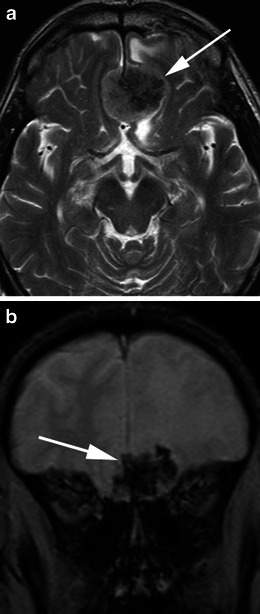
a Axial T2-weighted sequence in a 75-year-old man being investigated for Bell palsy demonstrates a heterogeneous, extra axial mass in the midline of the olfactory groove, left more than right, in keeping with a meningioma (*arrow*) associated with mild oedema within the left frontal lobe. There are intrinsic areas of low signal intensity in keeping with calcification that do not enhance on post contrast sequences. b Coronal T2* sequence in the same patient as a demonstrates susceptibility effect (*arrow*) within the mass in keeping with the presence of calcification
